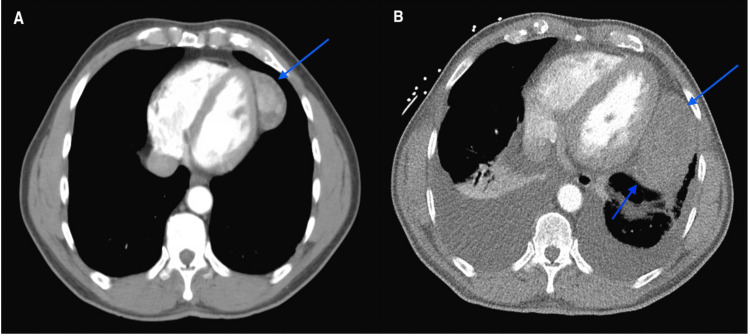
CT scan of the chest with IV contrast. (A) Mediastinal mass indicated by a blue arrow. (B) Nine-year interval mass growth delineated by blue arrows along with new pleural effusions. CT: computed tomography; IV: intravenous.
